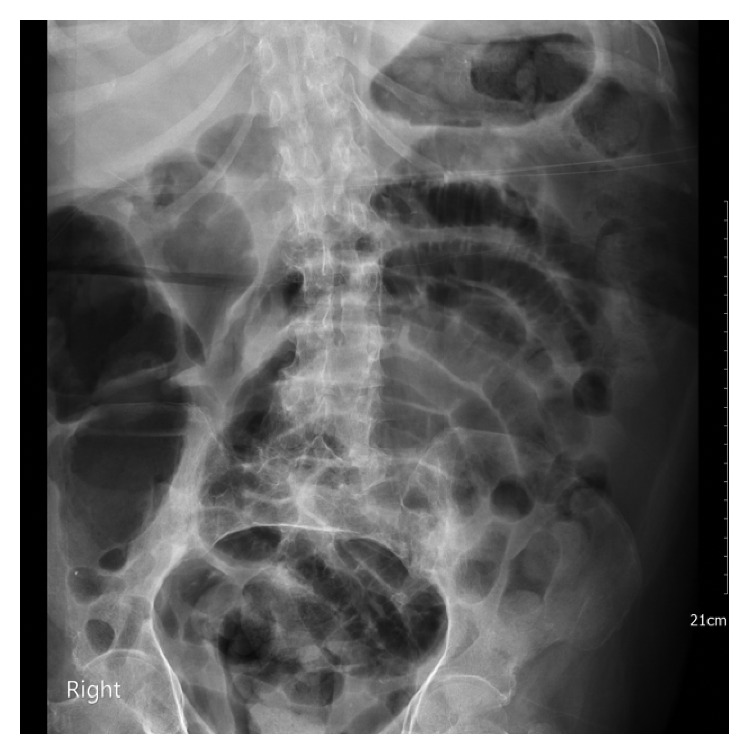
Diffuse gaseous distention of the bowel consistent with ileus seen on abdominal X-ray.
